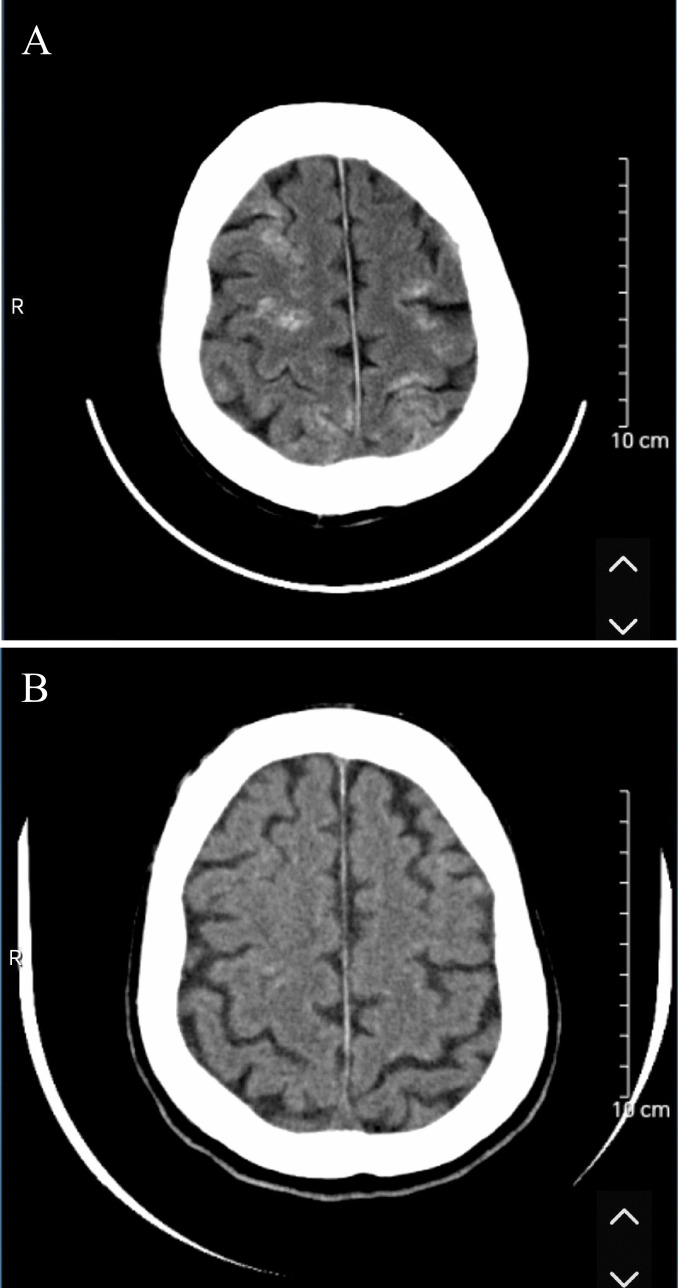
Head CT findings of cerebral lipiodol embolism. (A) One day after TACE: noncontrast cranial CT revealed multiple patchy hyperdense foci in the bilateral cerebral and cerebellar hemispheres. (B) One month after TACE: follow-up cranial CT demonstrated marked regression of the parenchymal hyperdense foci compared with the previous imaging.
